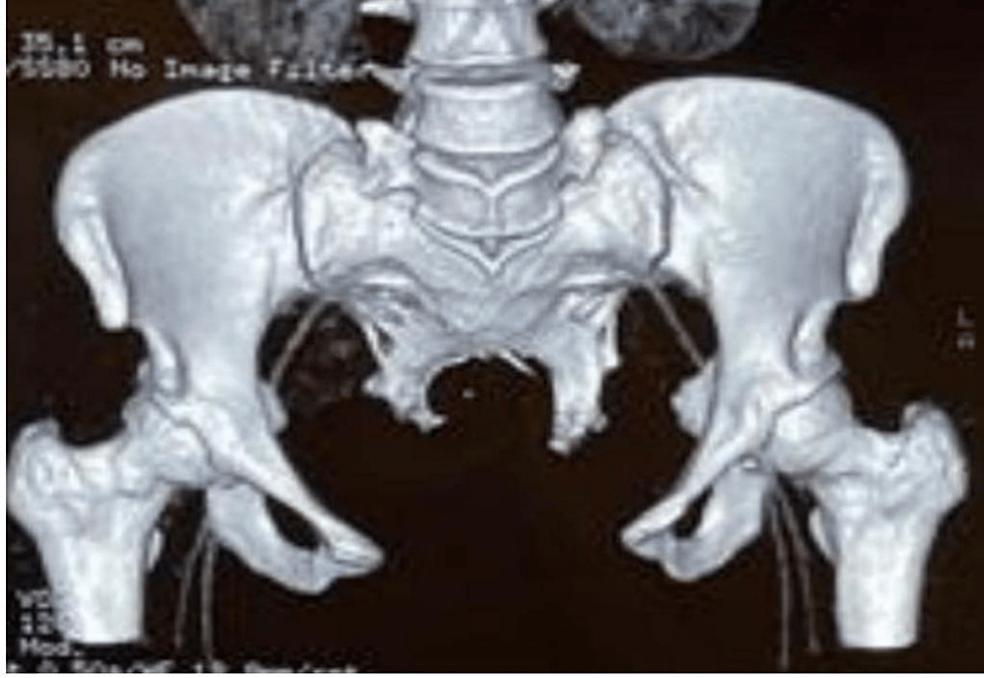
3D CT scan showing segmentation of first sacral vertebra, duplication of sacrum and pubic diastasis CT: computed tomography
